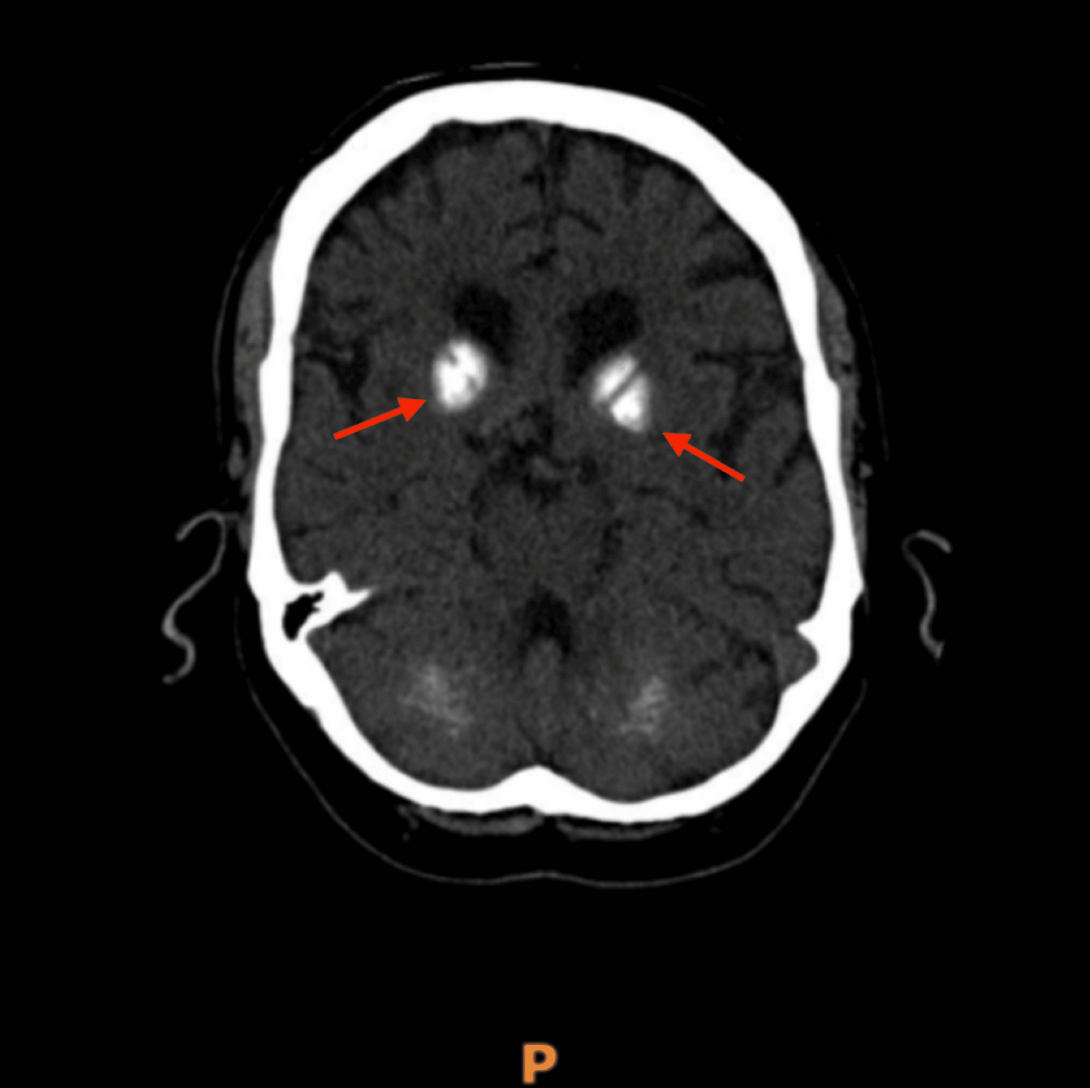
Axial non-contrast CT brain showing symmetric dense calcification in the bilateral basal ganglia (arrows), characteristic of Fahr’s syndrome.
